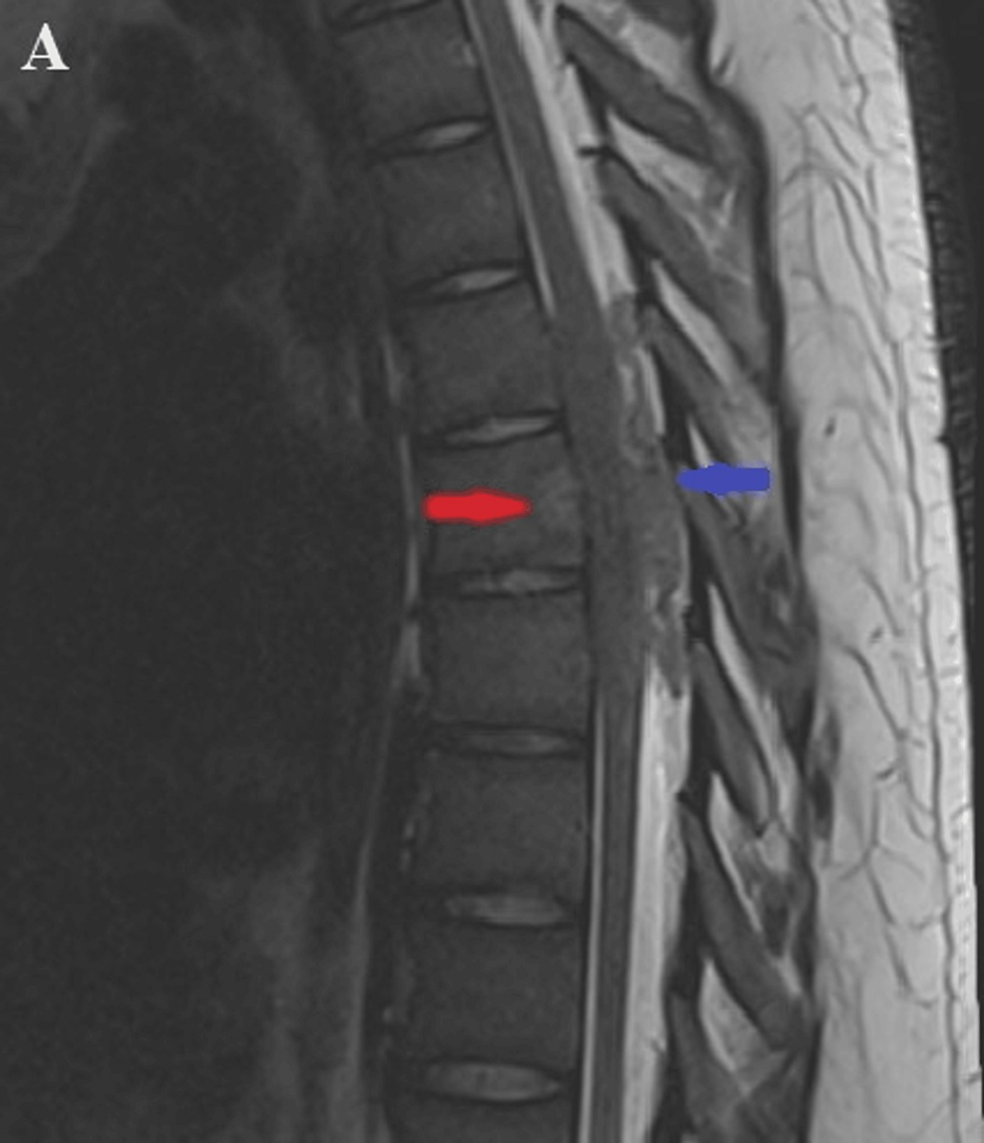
MRI of the thoracic spine in the sagittal and axial planes. The figure shows in T2 sequence isodense to hyperdense formation at the levels of TH5 to TH7 vertebral projections, a formation filling the posterolateral epidural space, compressing the adjacent spinal cord with local edema of the latter (blue arrow). Soft tissue destruction of the body of TH7 vertebra (red arrow).
